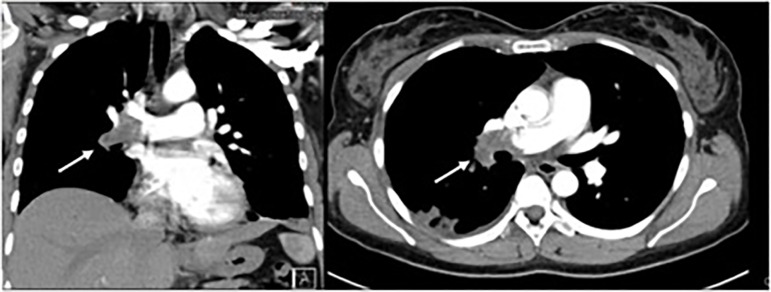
Chest computed tomography (CT): coronal section (left) and axial
